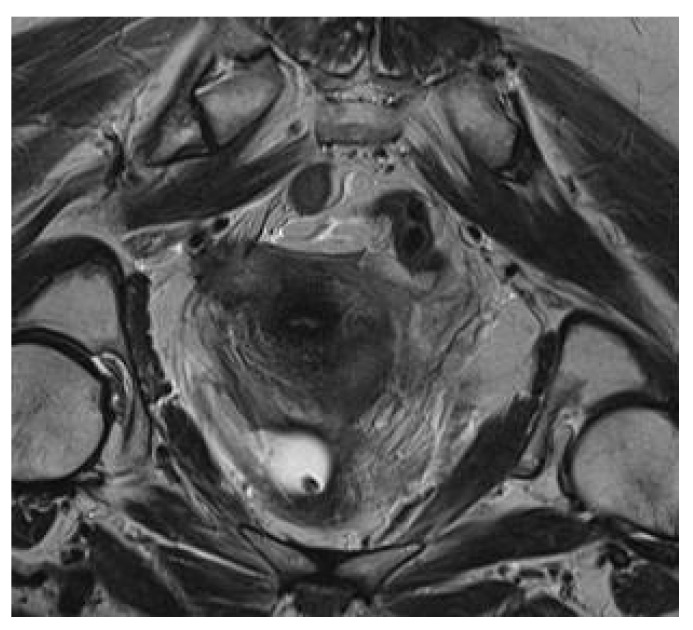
Pelvic magnetic resonance imaging (MRI) revealing the presence of a large tumoral nodule compressing the right ureter.
